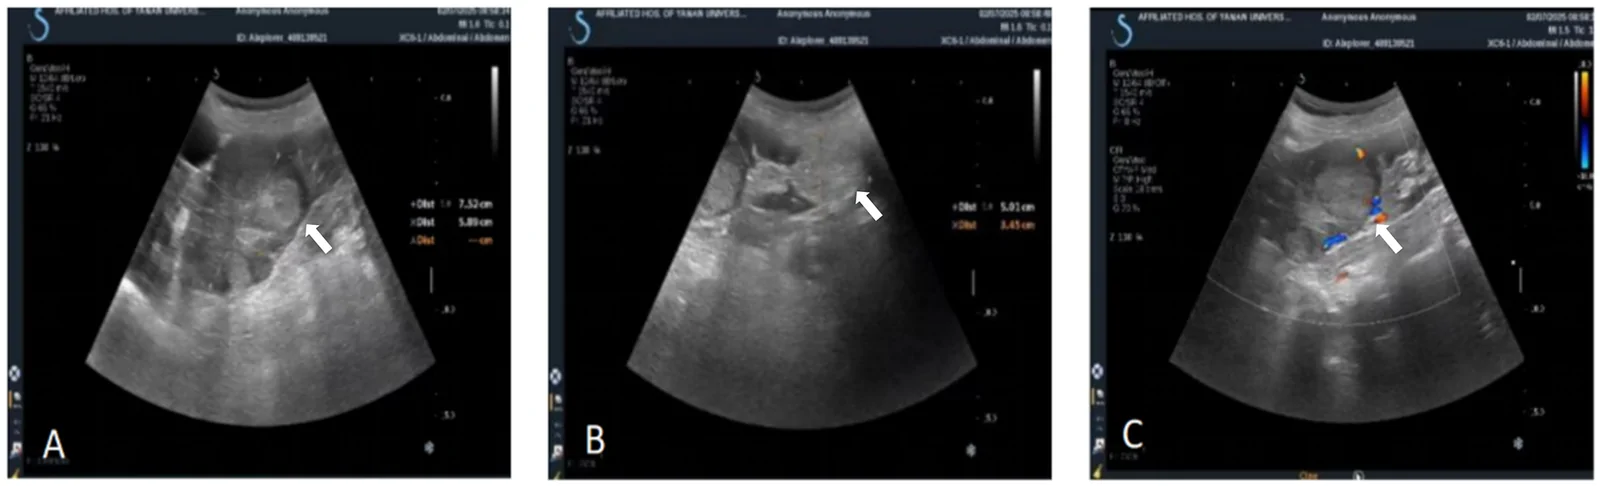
Renal ultrasonography. (A) Gray-scale ultrasonography shows a heterogeneous hypoechoic mass in the upper–mid pole of the right kidney (white arrows). (B) A second, slightly hyperechoic exophytic mass is seen in the lower pole of the right kidney (white arrows). (C) Color Doppler flow imaging demonstrates peripheral annular vascularity with punctate/strip-like intralesional flow (white arrows).
